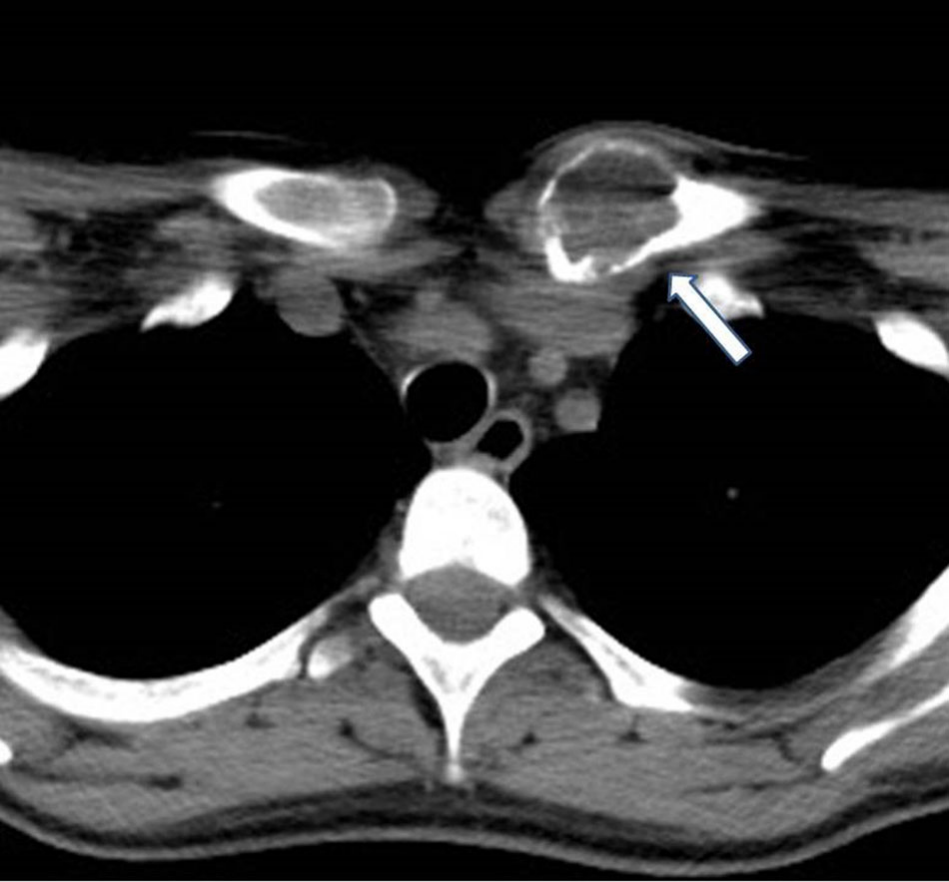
Case 1: CT scan—the similar osteolytic region of left clavicle (arrow)
